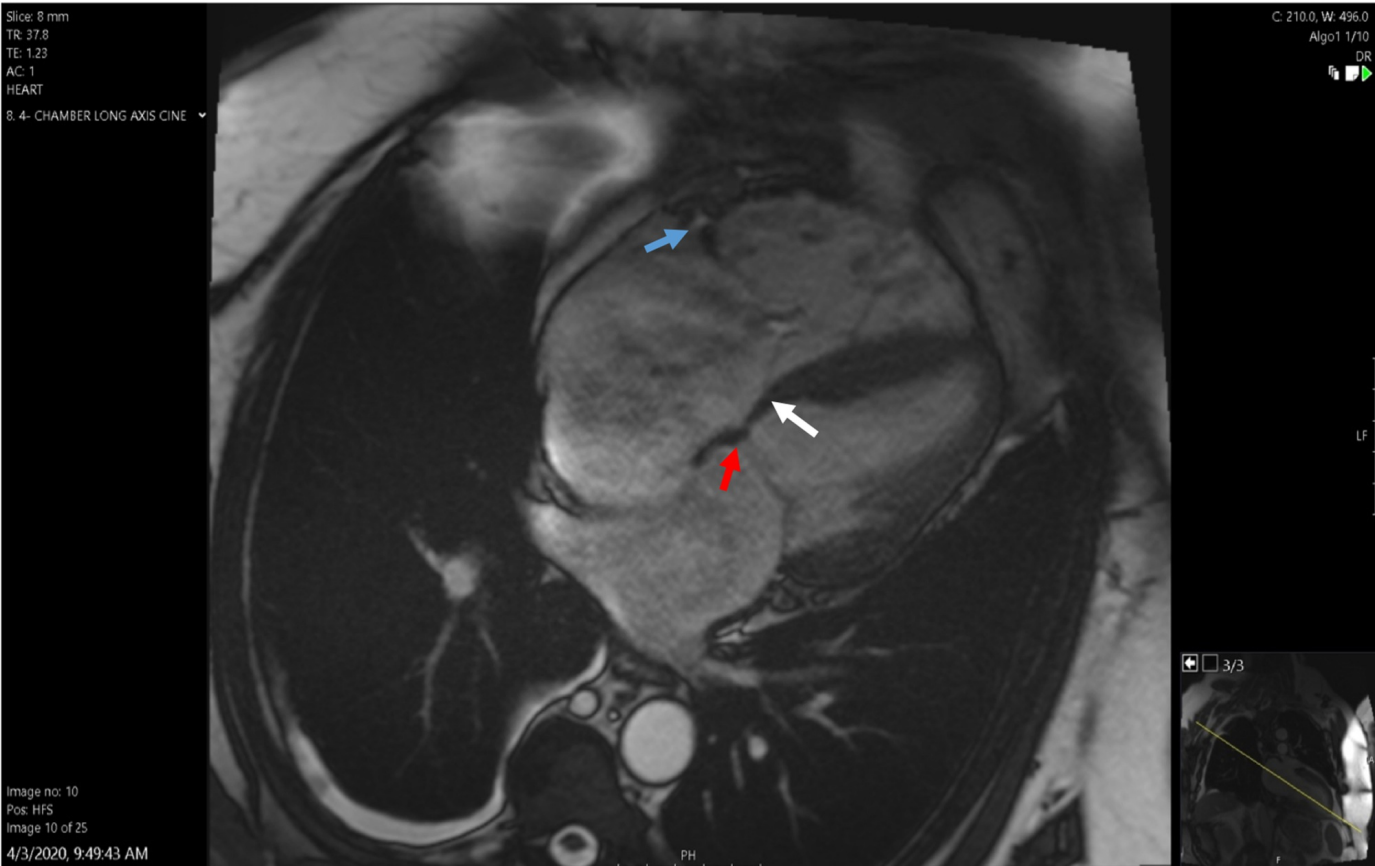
Cardiac MRI in four-chamber view showing the apically displaced septal tricuspid leaflet (white arrow) in relation to the mitral annulus (red arrow). Anterior tricuspid leaflet shows a normal annular insertion (blue arrow) but the coaptation point is lower in the right ventricle
